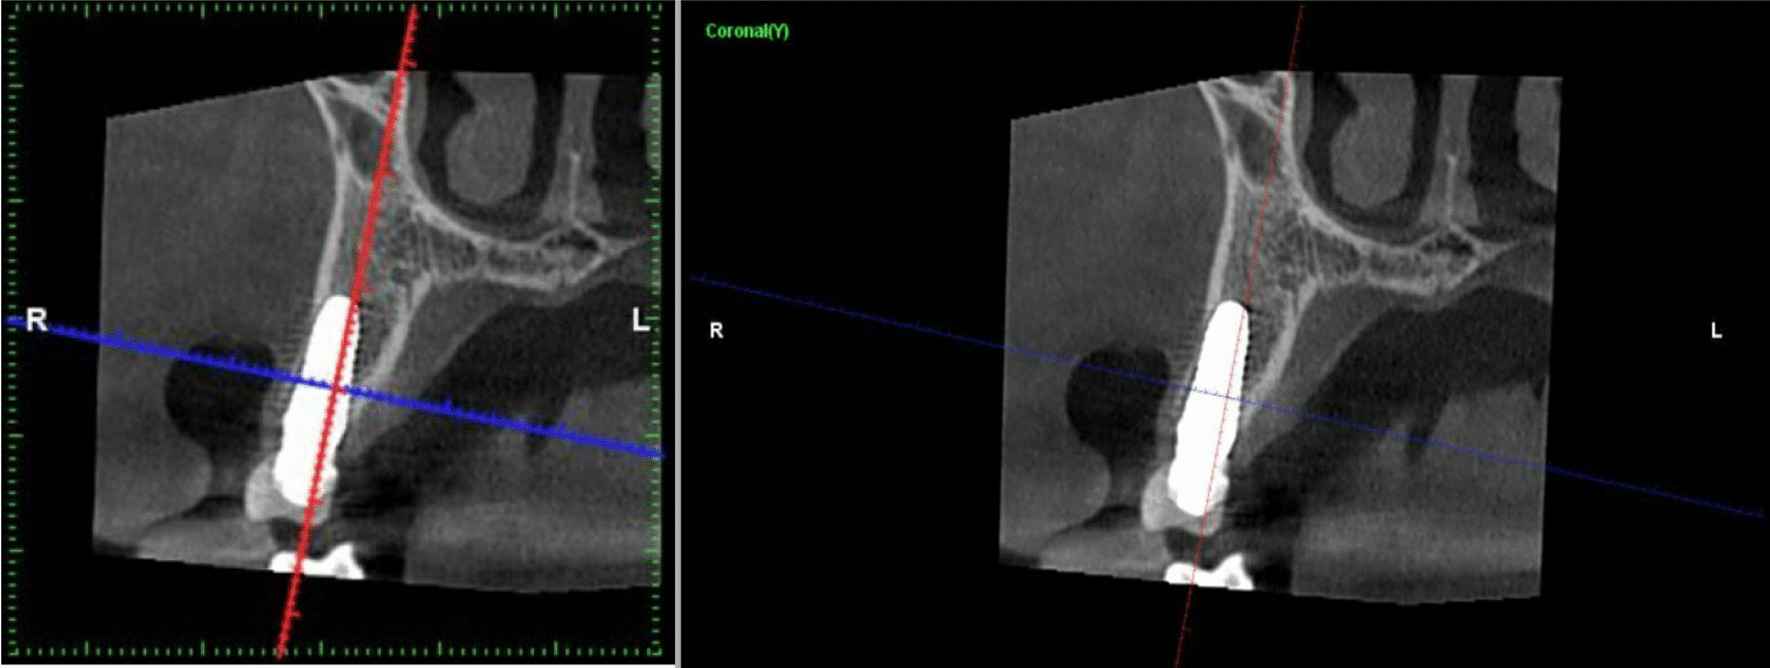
Computed tomography of zirconia implant after 5 years follow up
